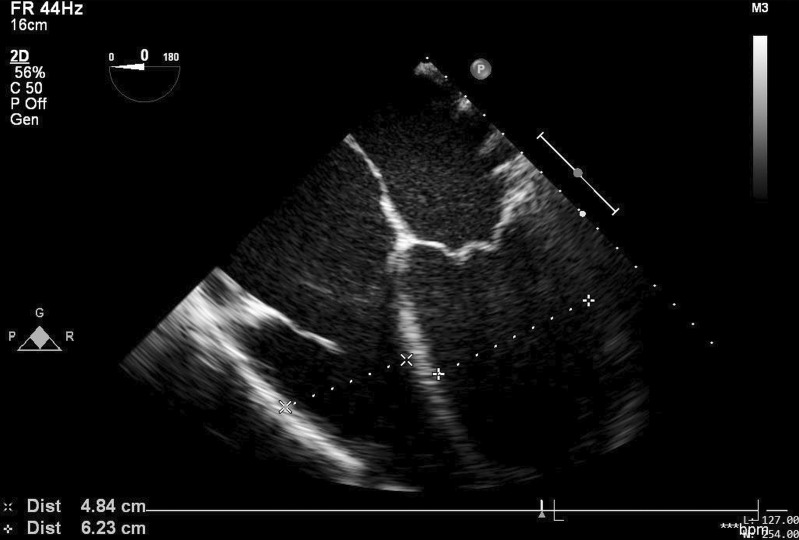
Initial transesophageal echo imaging demonstrating severe dilation of the right ventricle (diameter 4.84 cm) and left ventricle (diameter 6.23 cm) measured in four chambers view.
